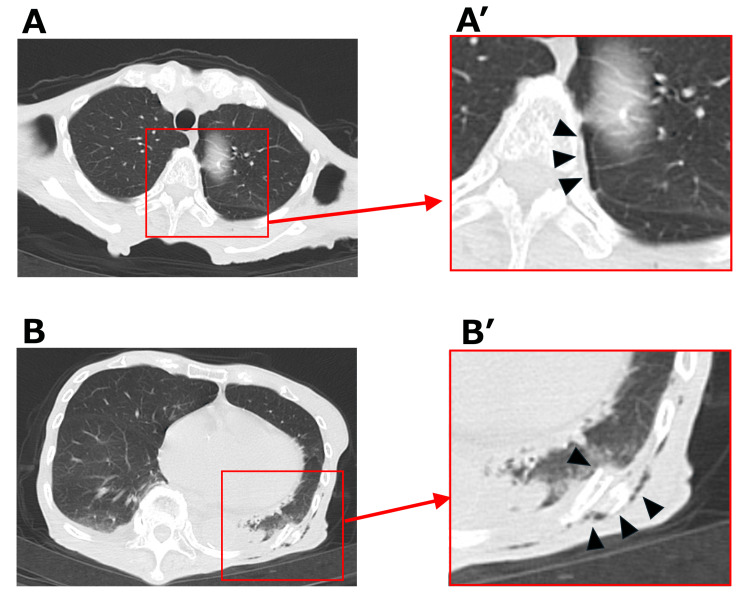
Axial CT on admission (Case 6) (A, A’) A minimal pneumothorax along the mediastinal surface is seen (black arrowheads). The pneumothorax measured <1 mm and was considered unsuitable for prophylactic chest tube placement because there was insufficient pleural air space for safe tube placement. (B, B’) At a different level, subcutaneous emphysema and displaced rib fractures with fragments projecting into the thoracic cavity are demonstrated (black arrowheads). CT, computed tomography
